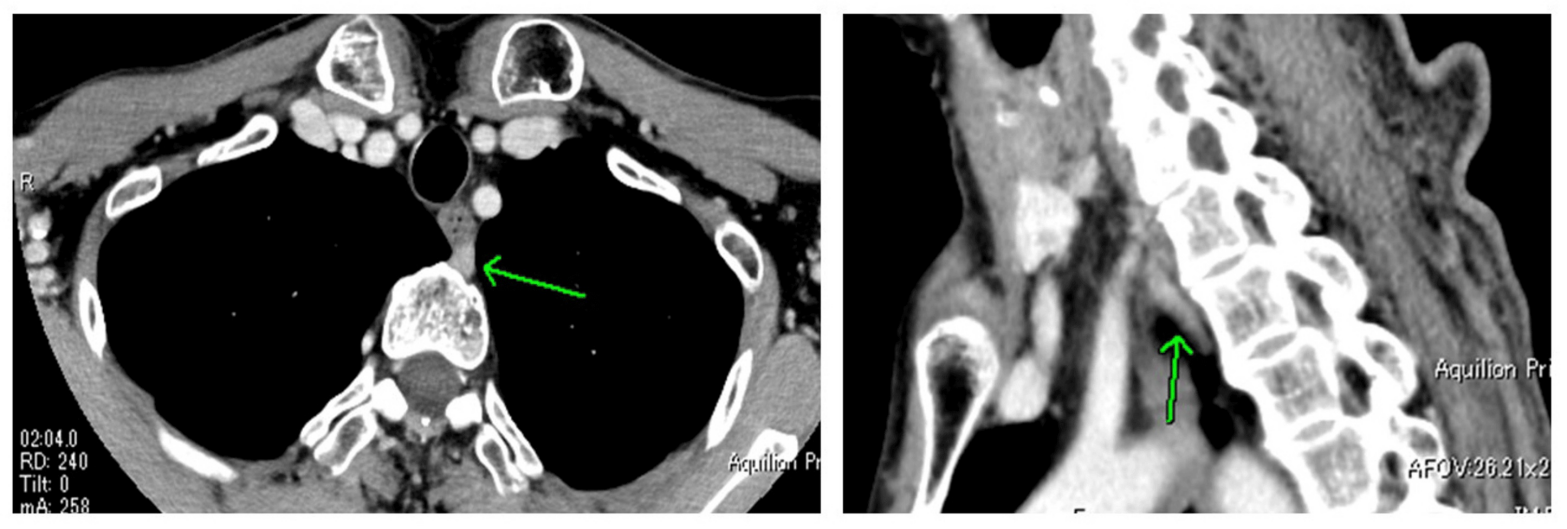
Contrast-enhanced CT Contrast-enhanced CT reveals a nodular mass with contrast enhancement between the anterior surface of the vertebral body at the Th1-2 level and the esophagus. There was no significant lymphadenopathy.
